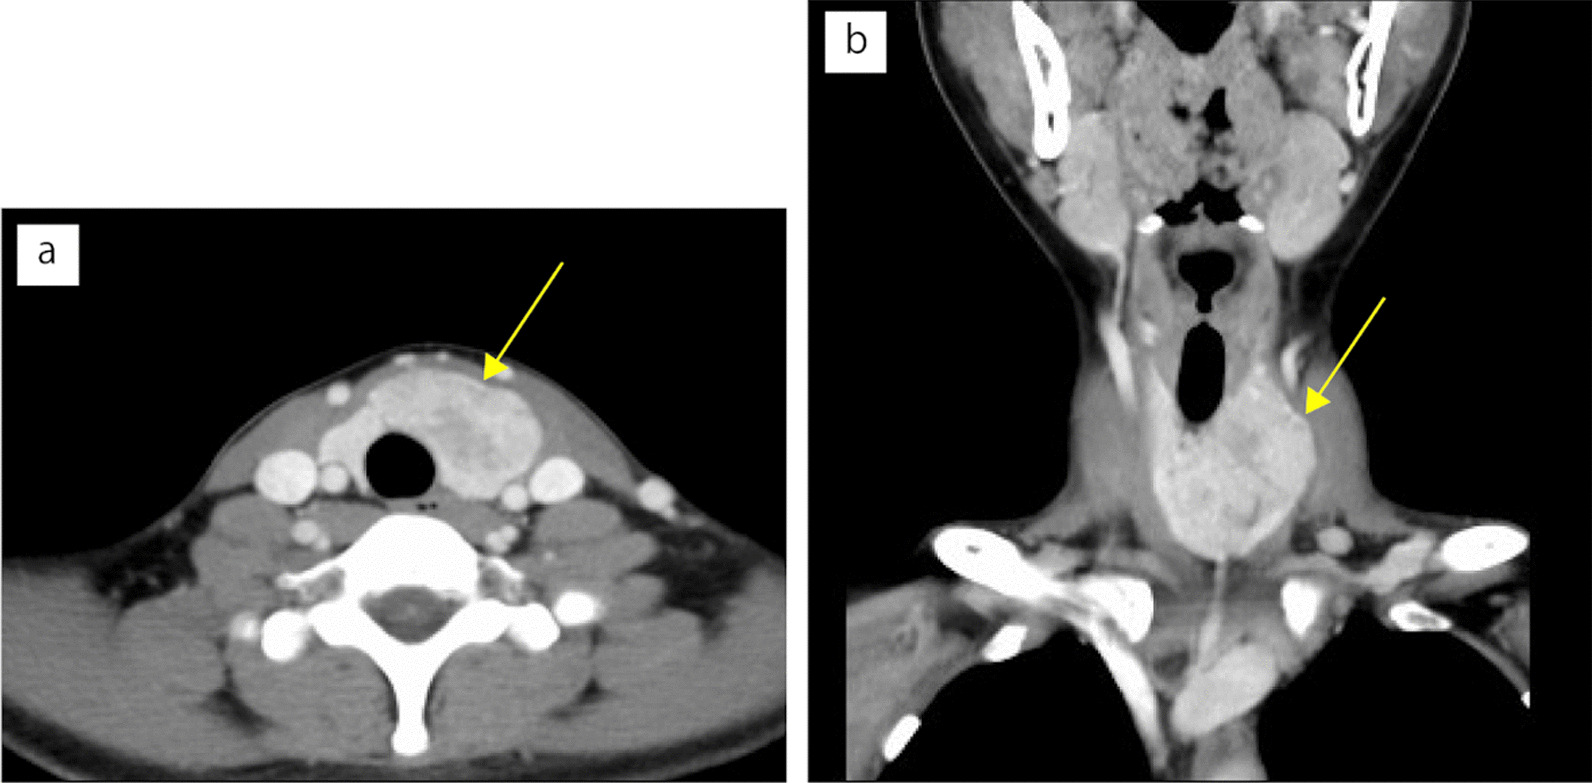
Contrast-enhanced computed tomography. a, b A nodular lesion (40 × 48 mm) is observed in lower pole to isthmus of the thyroid left lobe. The lesion showed heterogeneous enhancement. The arrows indicate thyroid tumor
